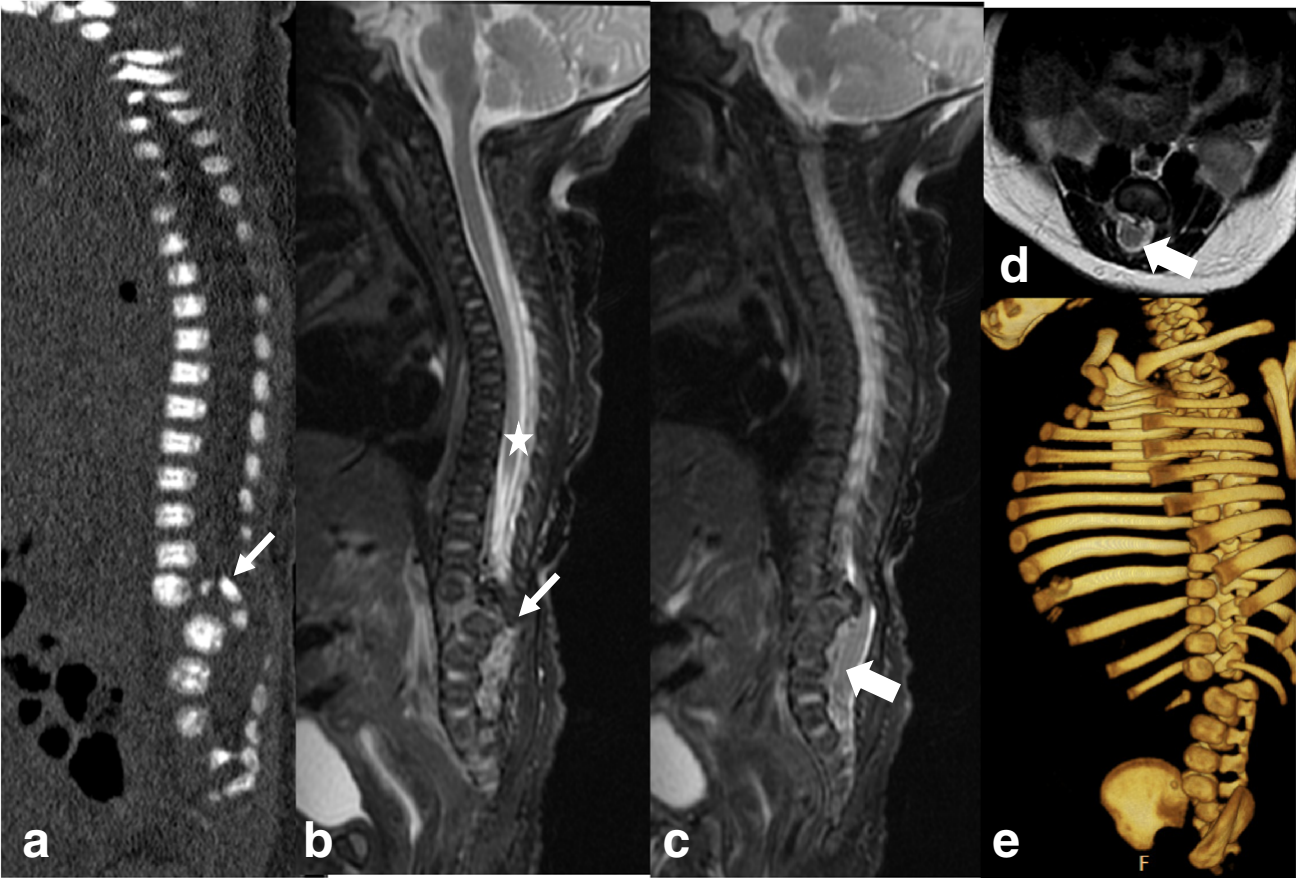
a-d: Segmental spinal dysgenesis in a neonate: Sagittal CT reconstruction (*a*) and sagittal T2 MR (*b*) images of the spine in the neonate with congenital paraplegia show absence of L1 and posterior dislocation of a hypoplastic L2 vertebra into the spinal canal (*arrows* on *a* and *b*). The spinal cord (*star* on *b*) is absent over the affected segments of the spine, but is thick and abnormally low further distally (*block arrows* on *c* and *d*). The 3D bony reformats from concomitantly performed CT (*e*) redemonstrate absence of L1 and hypoplasia of the posteriorly dislocated L2
